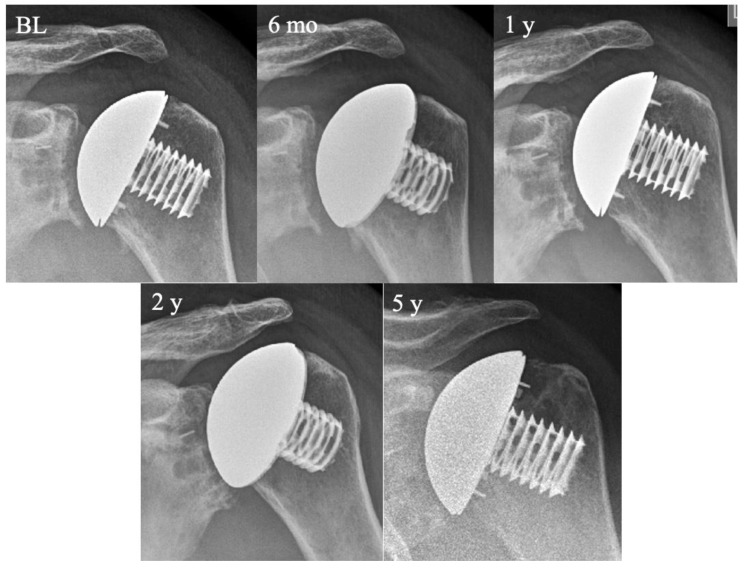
Progression of medialization and cranialization over time. Anteroposterior radiographs with the patient positioned in internal rotation. Images depict time points immediately after surgery (baseline, BL) and follow-ups after six months (6 mo) and one, two, and five years later (from top left to bottom right).
